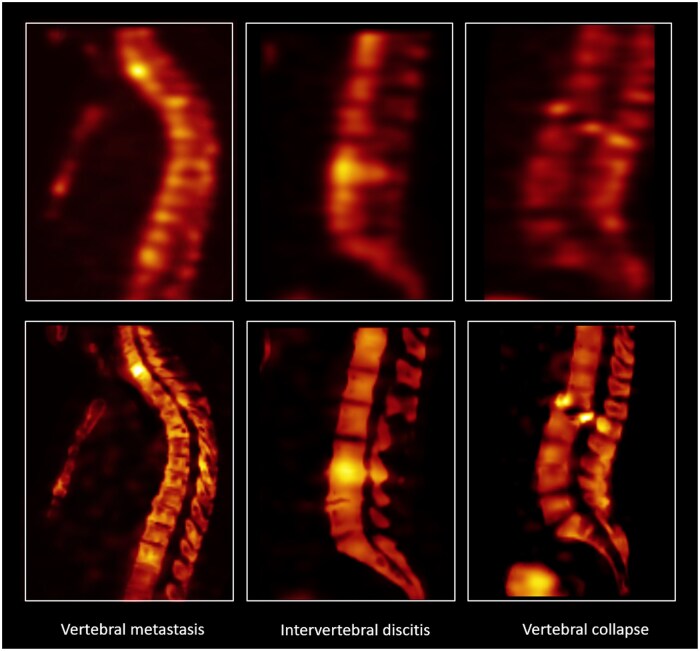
Figure 4. Example of OSCGM reconstruction with Siemens’ xSPECT bone algorithm compared to a standard bone scintigraphy reconstruction, demonstrating improved delineation of vertebral structure. Image provided by Siemens Healthineers, data courtesy of John Hopkins University, Baltimore, MD, USA Reference S119_22 (left), the University of Erlangen, Germany, Reference FAU_VS36 (middle) and Friedrich Alexander University, Erlangen, Germany Reference S157_21 (right).
